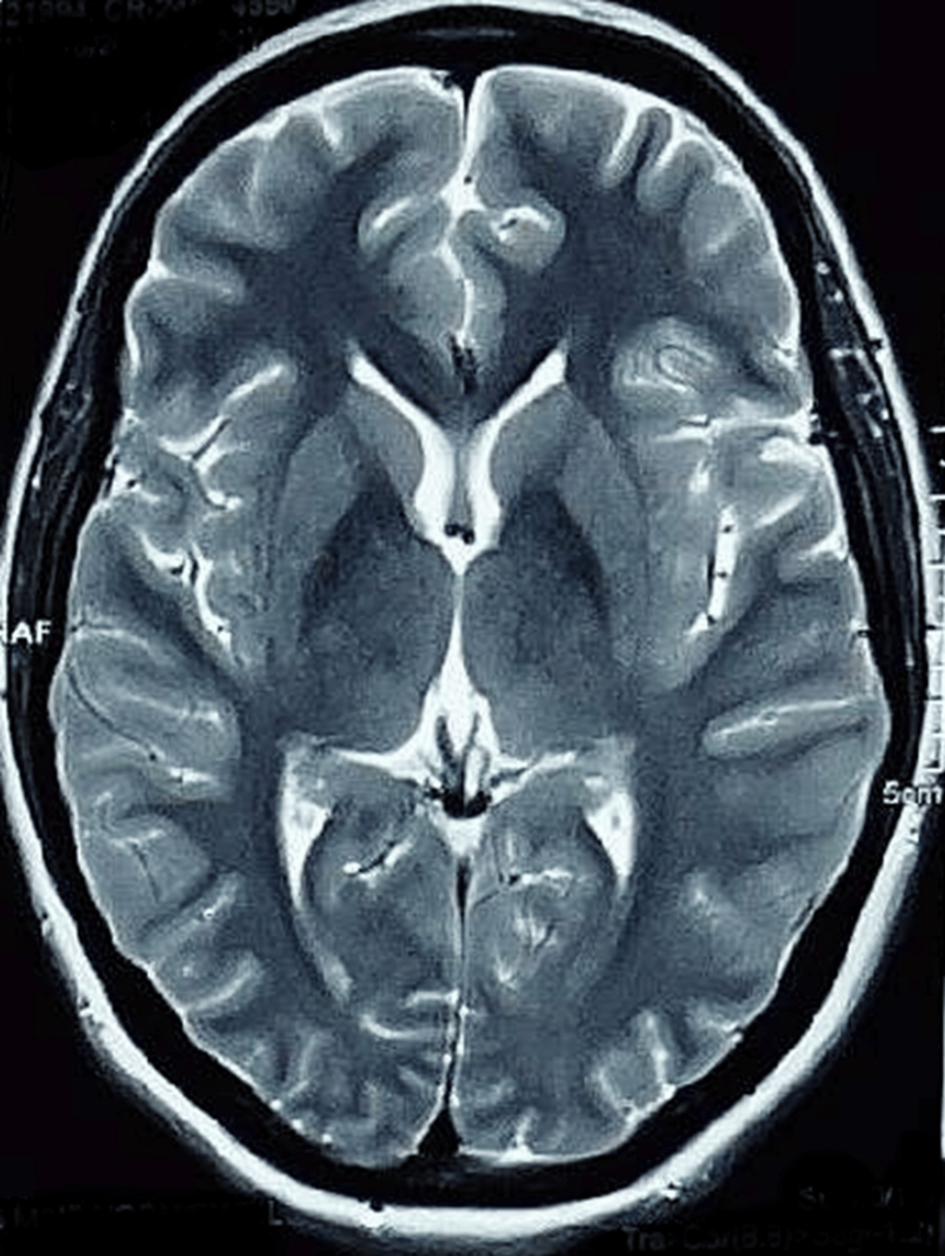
T2-weighed MRI brain sequence with normal results
